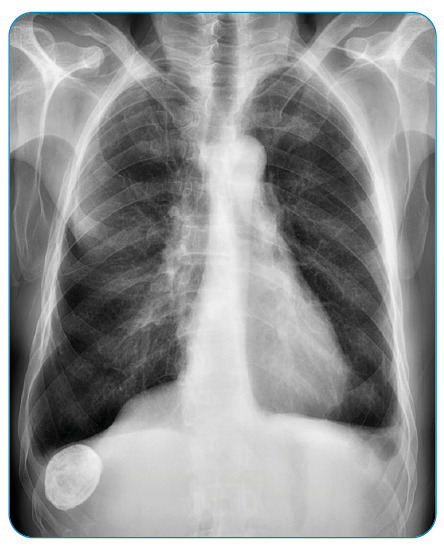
Posteroanterior chest radiography showing a subdiaphragmatic calcified hepatic hydatid cyst.
